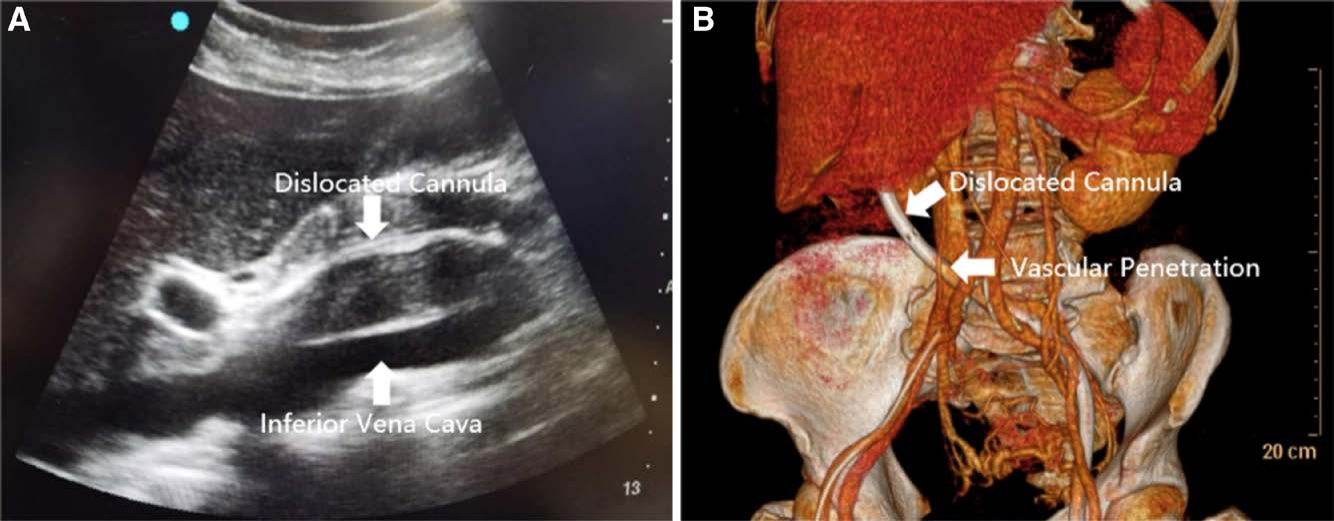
Abnormal position of the drainage cannula was detected upon imaging examinations. (A) Ultrasound showed that the cannula tip was not located at the entrance of the inferior vena cava in the right atrium, but close to the outer wall of the vessel. (B) Abdominal computed tomography venography (CTV) film with 3D reconstruction showing that the cannula tip perforated into the abdominal cavity from the conjunction of the iliac vein and inferior vena cava.
